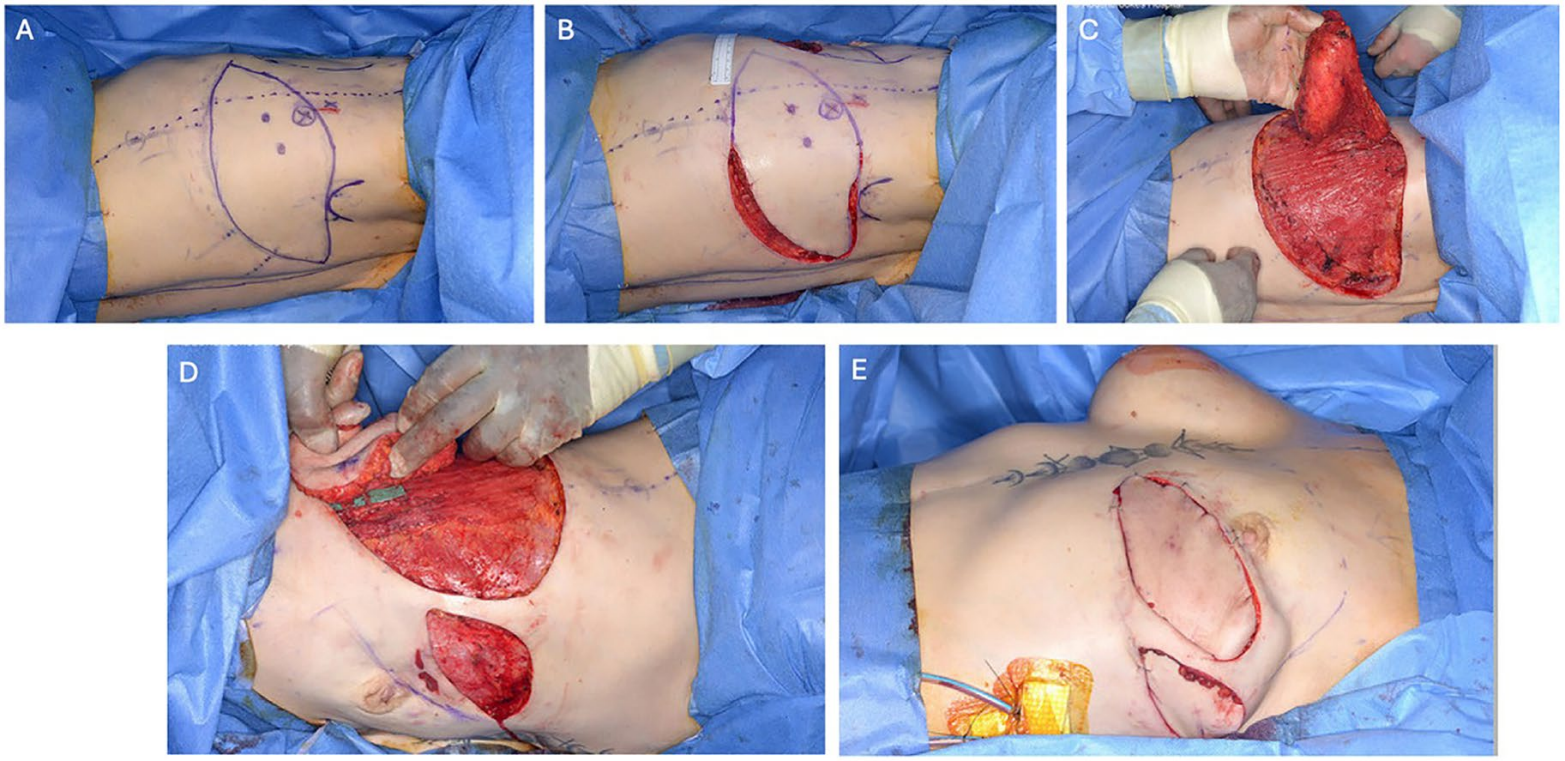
Intraoperative steps of thoracodorsal artery perforator (TDAP) flap harvest and inset. (A) Preoperative Doppler identification and marking of thoracodorsal artery perforators with the patient in the lateral decubitus position. (B) Incision and dissection of the skin paddle down to the muscle fascia. (C) Elevation of the flap from medial to lateral and superior to inferior to expose the dominant perforator. (D) Careful intramuscular dissection of the perforator through the latissimus dorsi muscle with division of side branches. (E) Final flap inset after subpectoral pocket creation, 180° rotation, and tunnelling beneath the split latissimus dorsi muscle.
